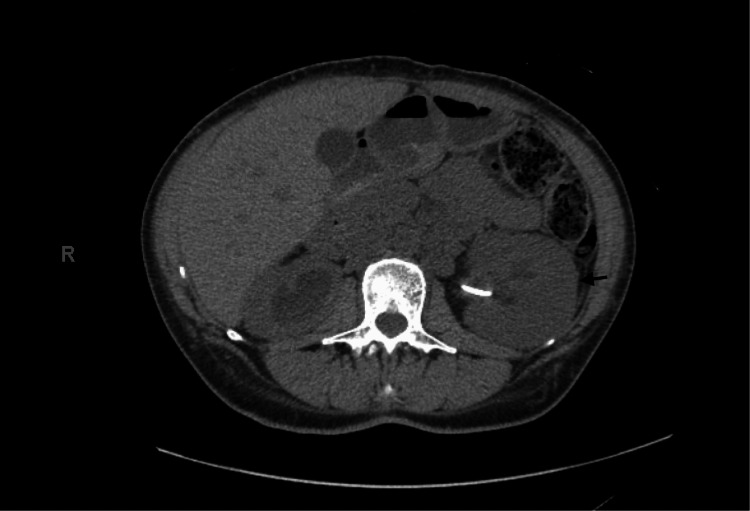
Non-contrast axial CT of abdomen and pelvis showing improvement in bilateral emphysematous pyelonephritis after one week of intravenous isavuconazole.
